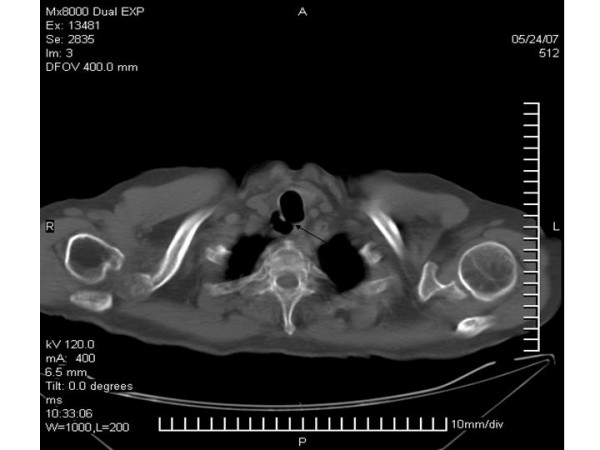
On CT scan the diverticulosis is demonstrating on the right posterior-lateral region of the trachea. The connection with the tracheal lumen is also obvious at the posterior wall of the trachea.
